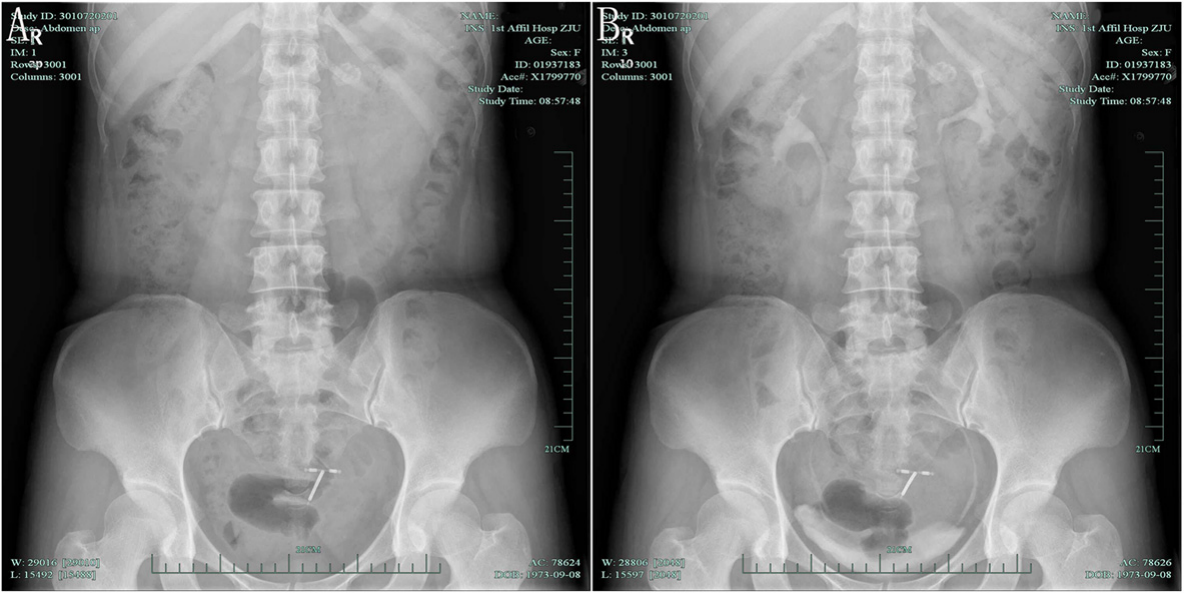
The images from the abdominal plain film and intravenous pyelography. a An abdominal plain film showed a left-sided renal calcification. b After injecting contrast medium, intravenous pyelography showed normal renal pelvis and a patchy shadow within the left kidney contour
